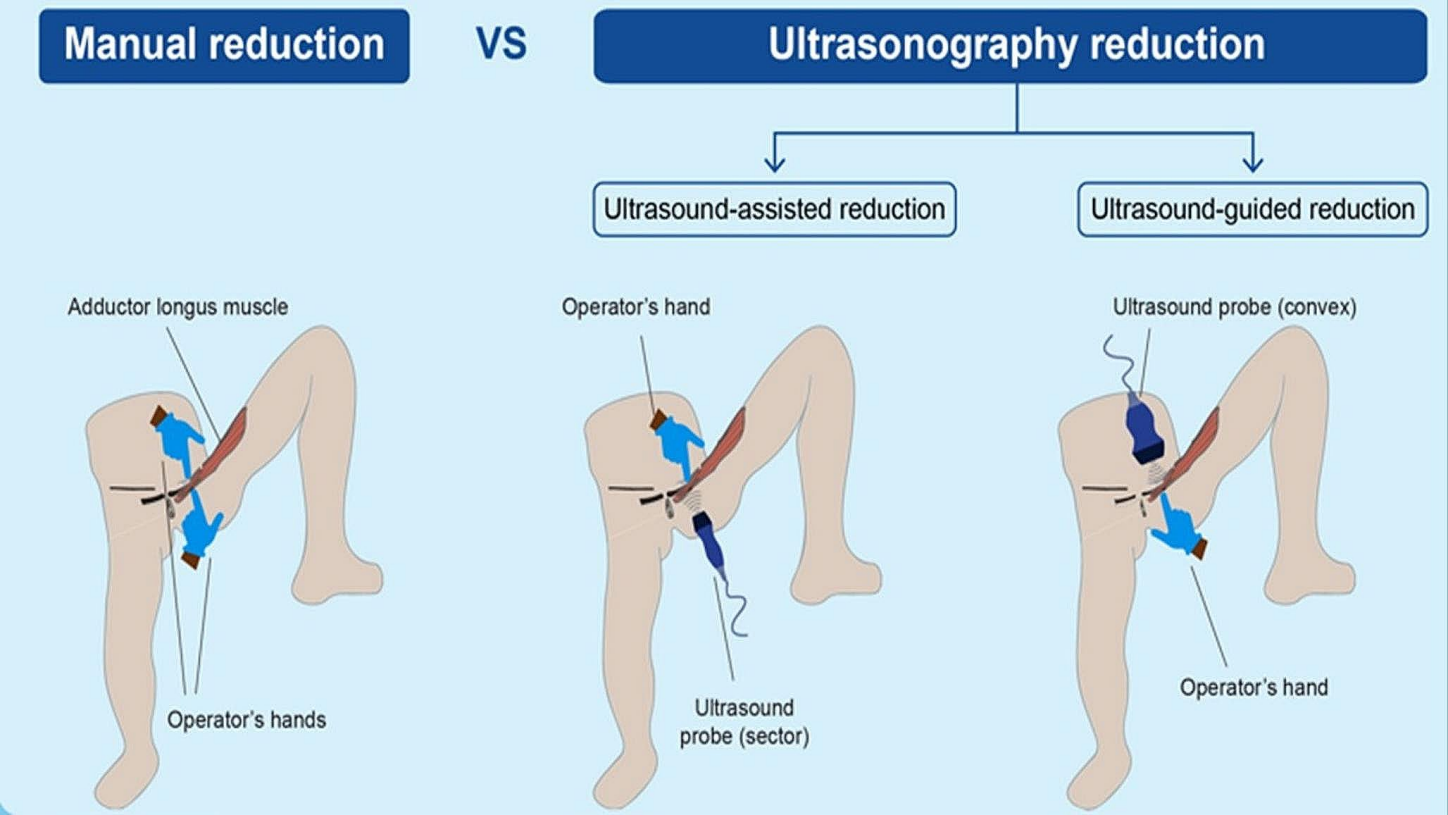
Ultrasound-assisted and ultrasound-guided reduction methods
